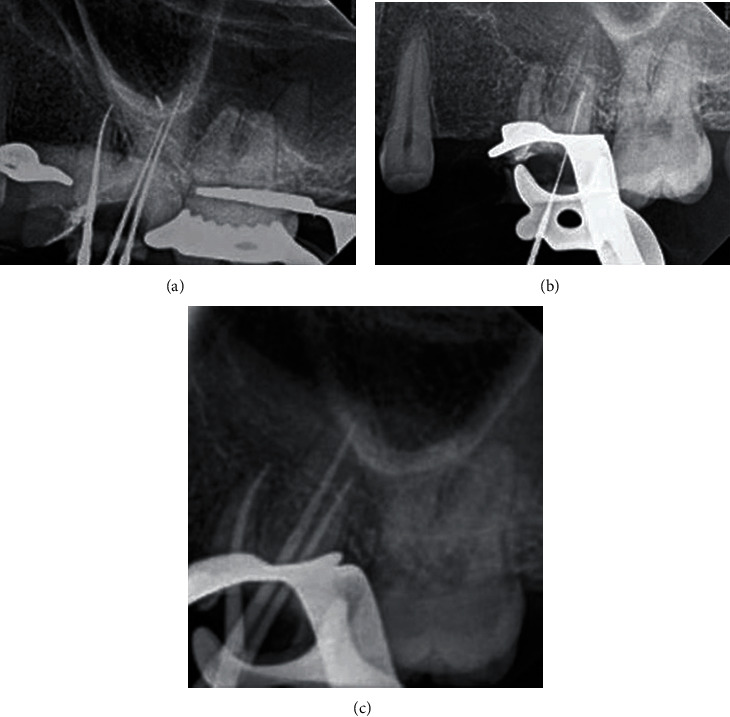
Intraoral periapical radiograph for the treatment procedure of maxillary left first molar. Working length was determined (a). Placement of MTA to repair the perforation site at the distobuccal root (b). Matching cone for the three canals after cleaning and shaping was done (c).
